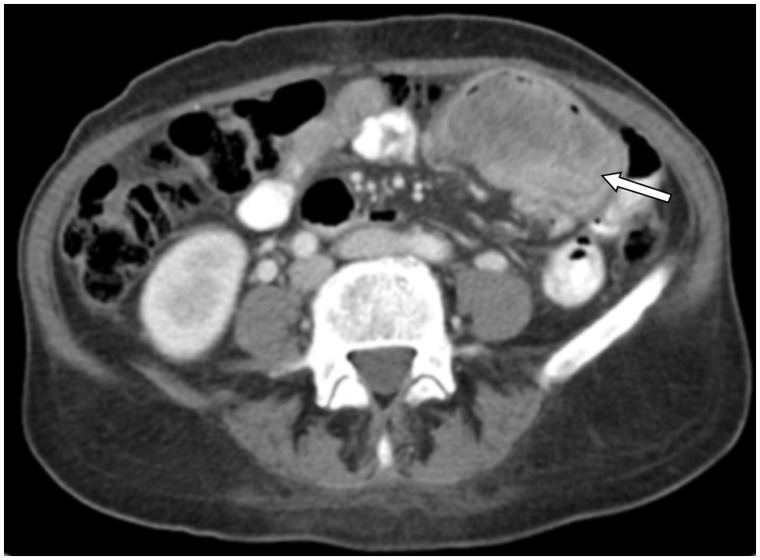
Abdominal CT scan showing a mass arising from the small bowel.
